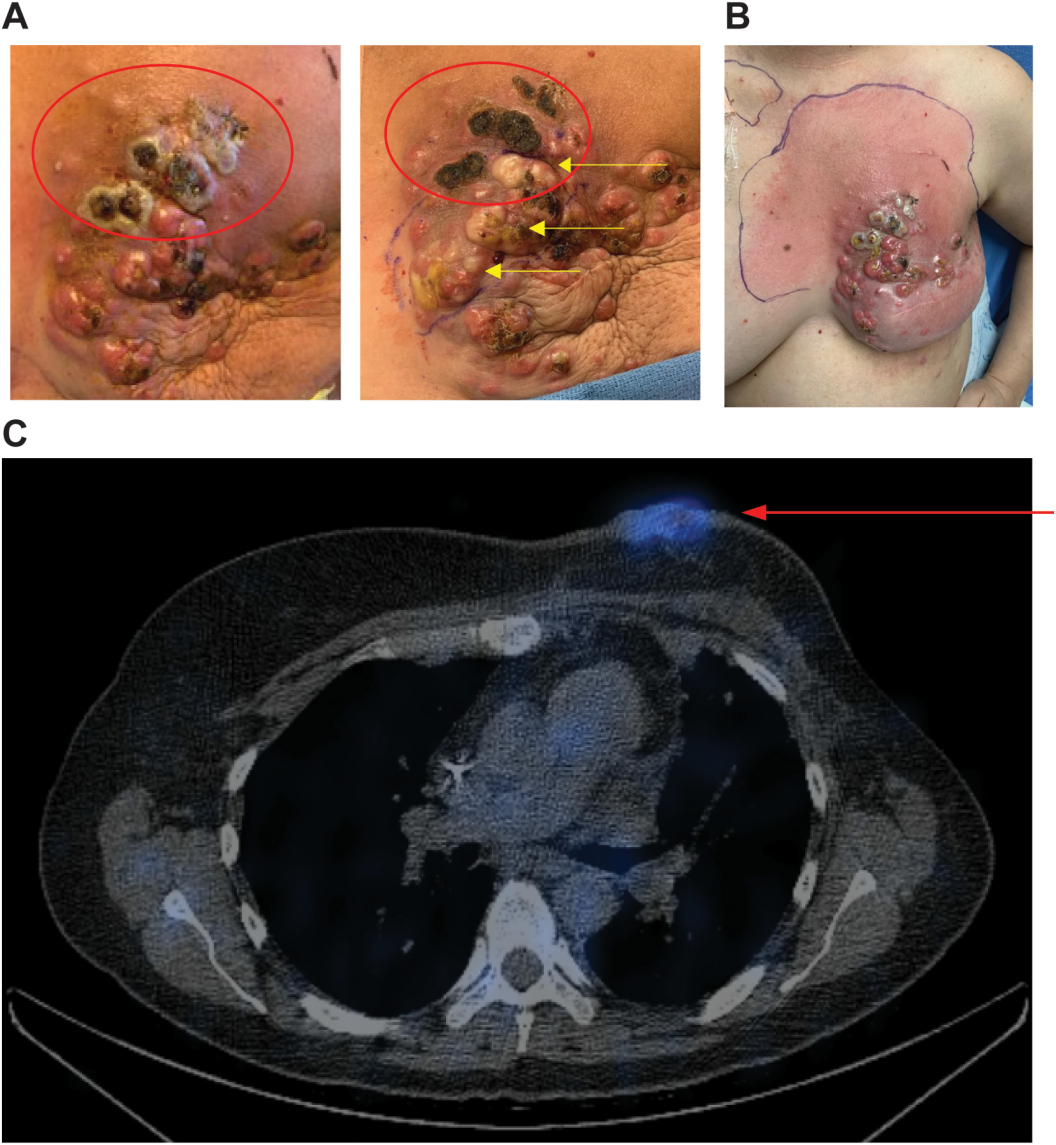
Robust injection site reaction and uptake on SPECT imaging following treatment with dose level 3. (A) Patient 2 received CF33-hNIS-antiPDL1 at dose level 3 (1x10^6^ PFU). Injected lesions were superior left chest wall nodules. Injected nodules demonstrated necrosis on clinical exam (red ovals). The image on the left shows C1D11, right shows C1D21 with continued necrosis of superior injected lesions and blanching of lesions inferior to that following injection #2 into the inferior lesions (yellow arrows). (B) Robust injection site reaction shown on C1D8 with surrounding erythema. (C) SPECT imaging showed enhancement of injected nodules (red arrow).
